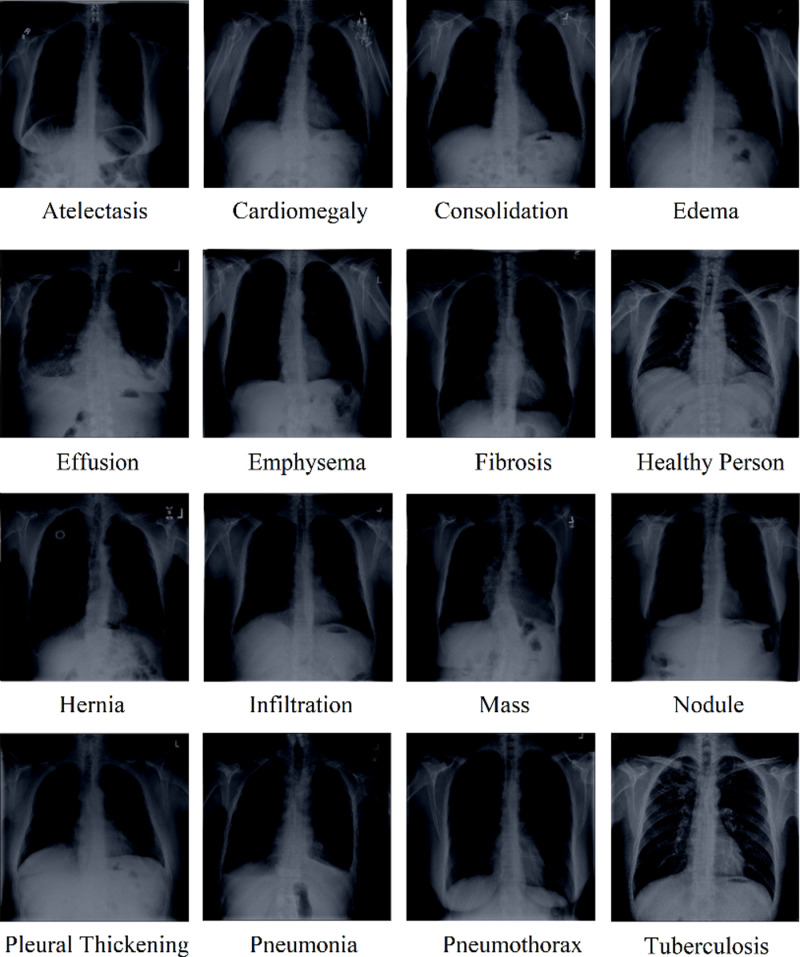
Representative chest radiographs of all classes after preprocessing.
